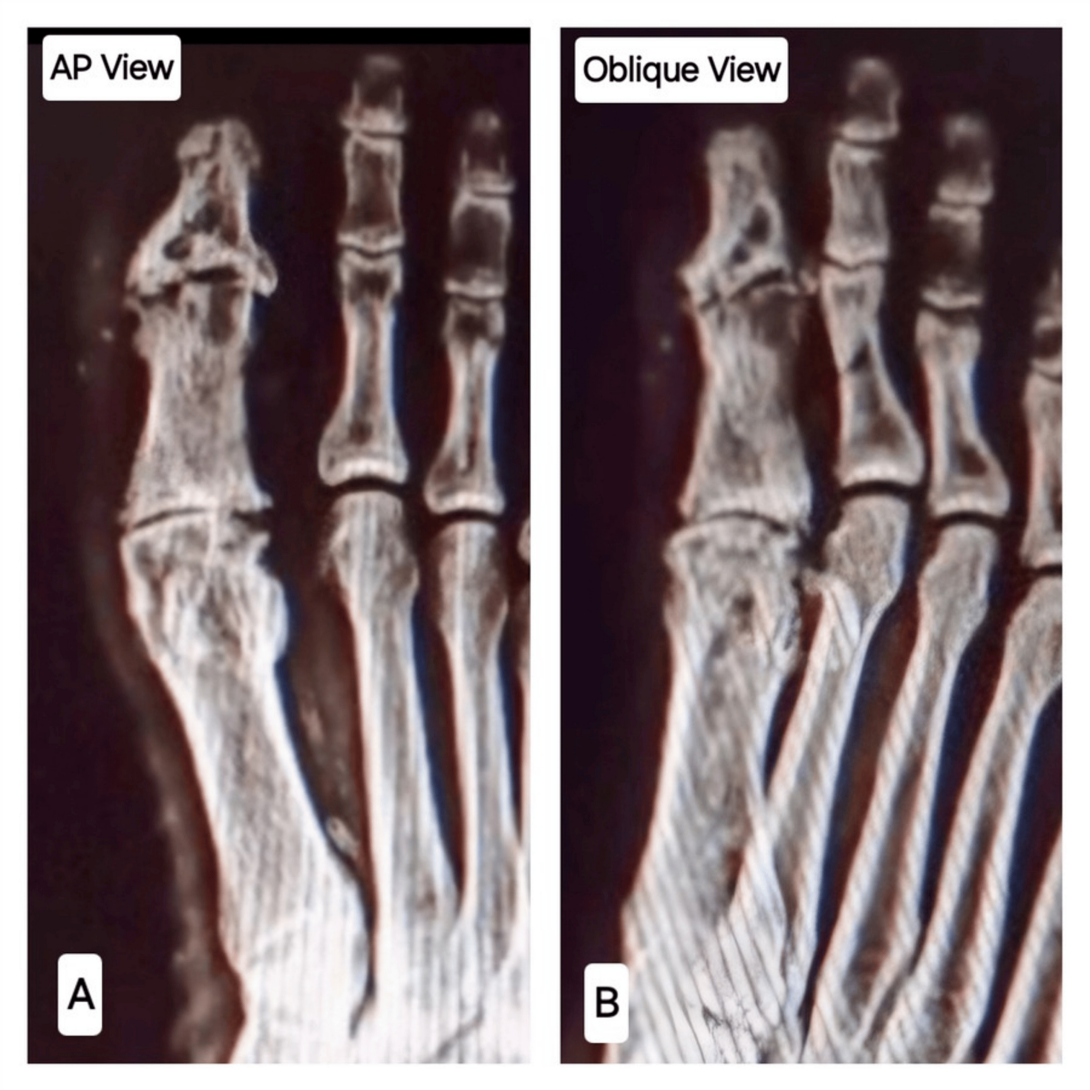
Plain radiograph of the affected toe (post-operative at six months follow-up)
